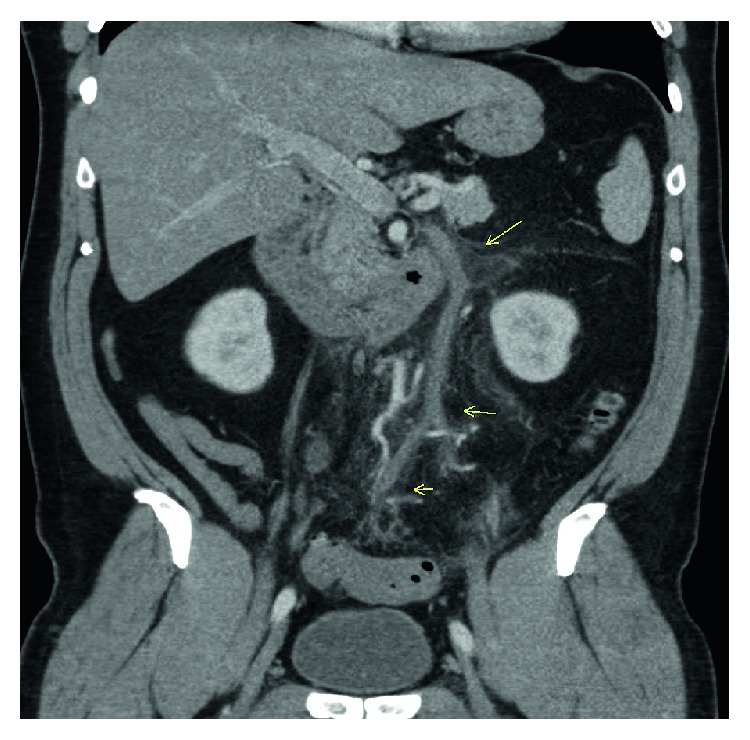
CT abdomen showing induration of the mesentery along the entire course of the inferior mesenteric vein with partial filling defects (marked with arrows).
